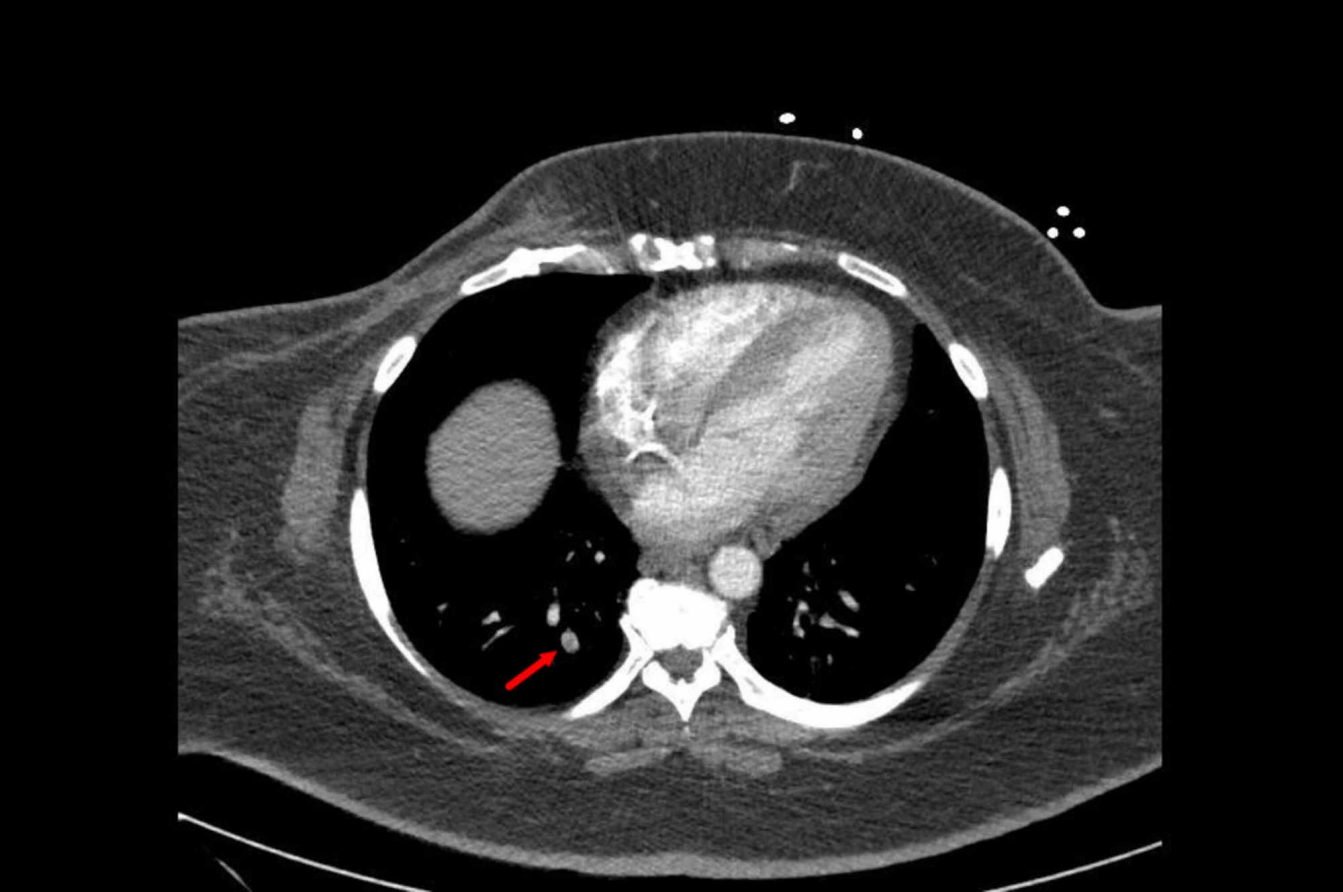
CT scan showing a small right lower lobe pulmonary embolus.
